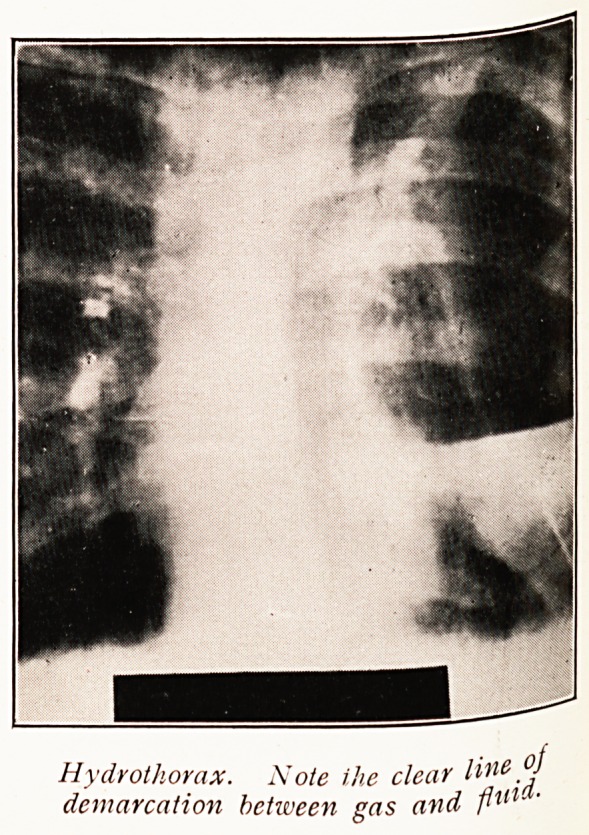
# Some Problems in Pulmonary Disease, with Special Reference to Radiography
